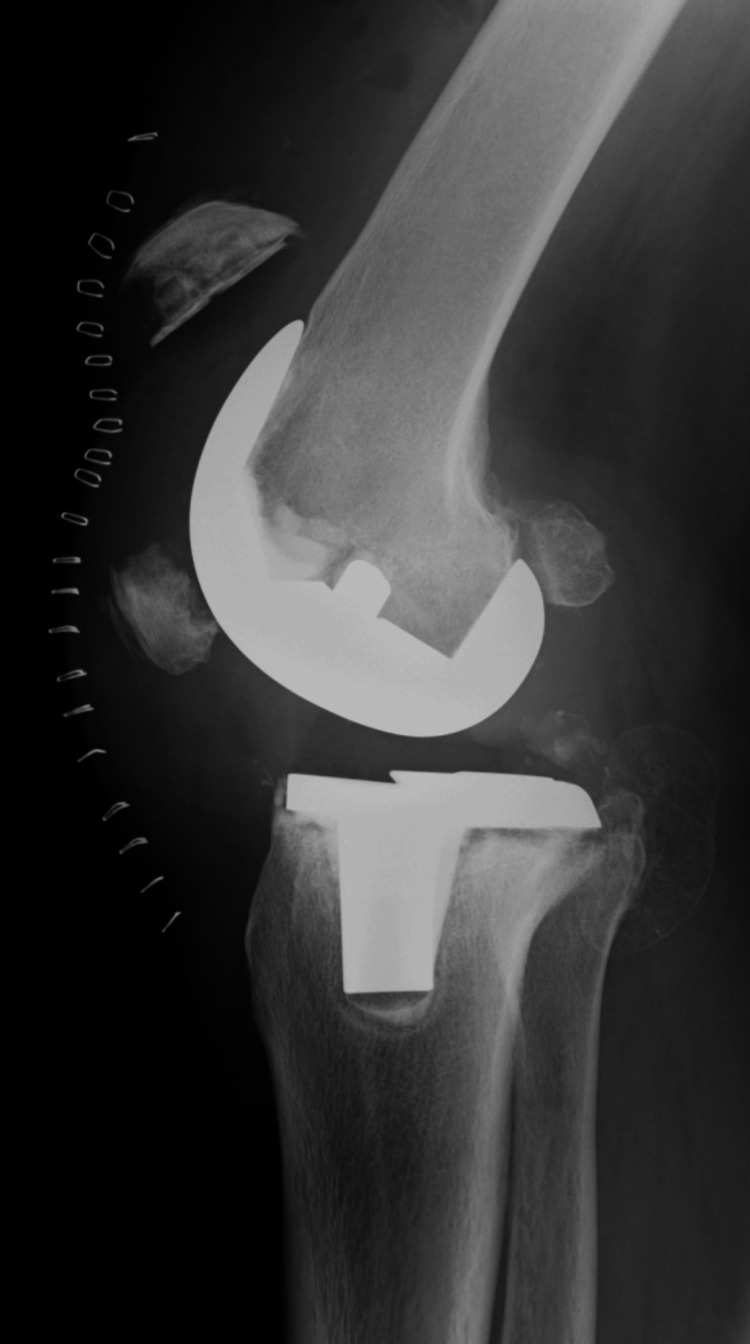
X-rays at the time of redisplacement of the fracture The X-ray revealed a redisplacement of the right patellar fracture.
